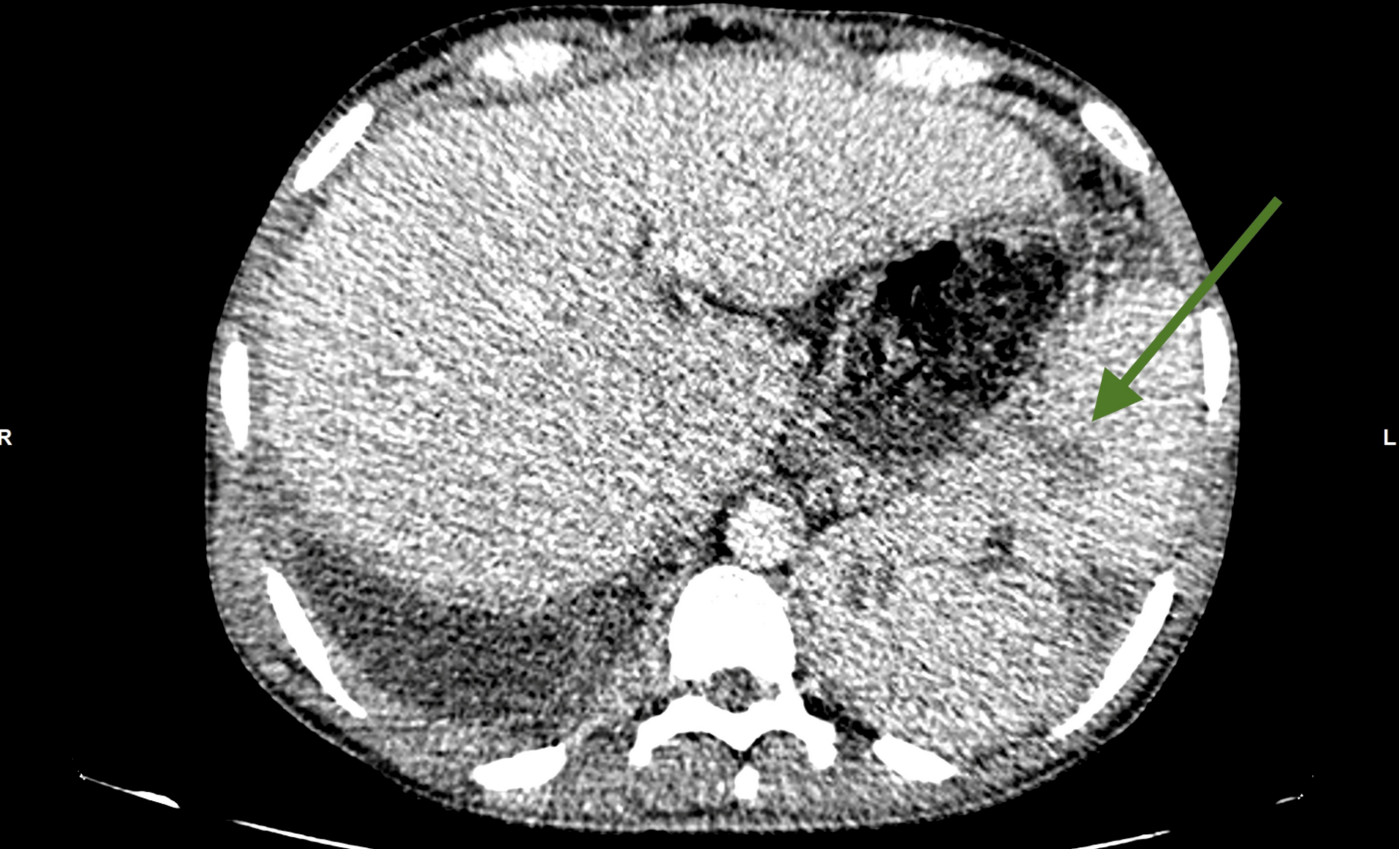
CT Neck and Trunk, axial view, showing hepatosplenomegaly. Green arrow shows splenic nodules
